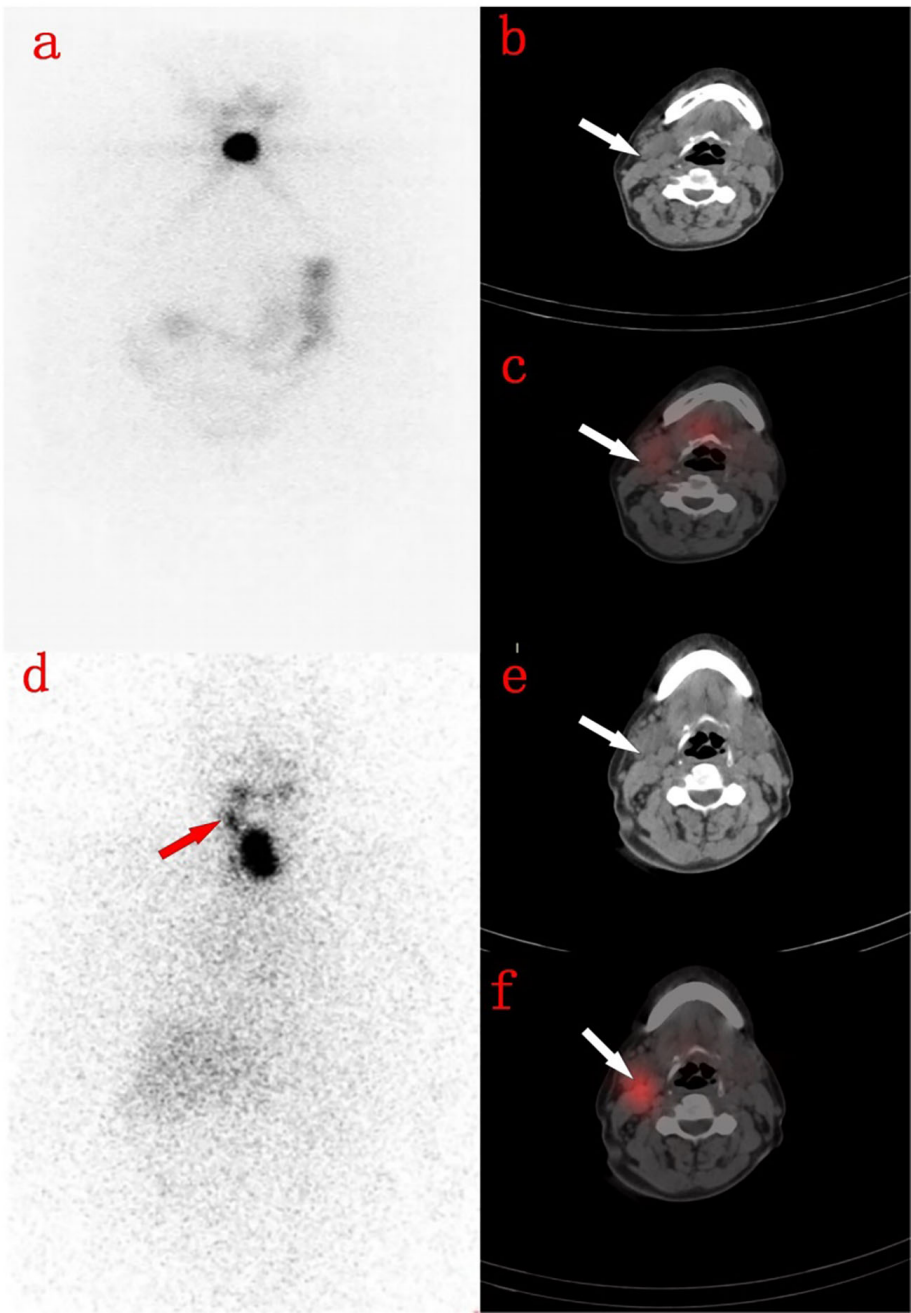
A 71-year-old woman with thyroidectomy 4 months ago. (A) shows the residual thyroid on the day 3 imaging. (B) shows the right cervical lymph nodes on the neck CT image. (C) shows the right cervical lymph nodes without radioactive uptake on the 3rd day SPECT/CT fusion image. (D) shows residual thyroid on the 7th day WBS, with radioactive accumulation in the right neck. (E) shows the right neck lymph nodes on the neck CT image. (F) shows the right neck lymph nodes showed radioactive accumulation on the 7th day SPECT/CT fusion image.
